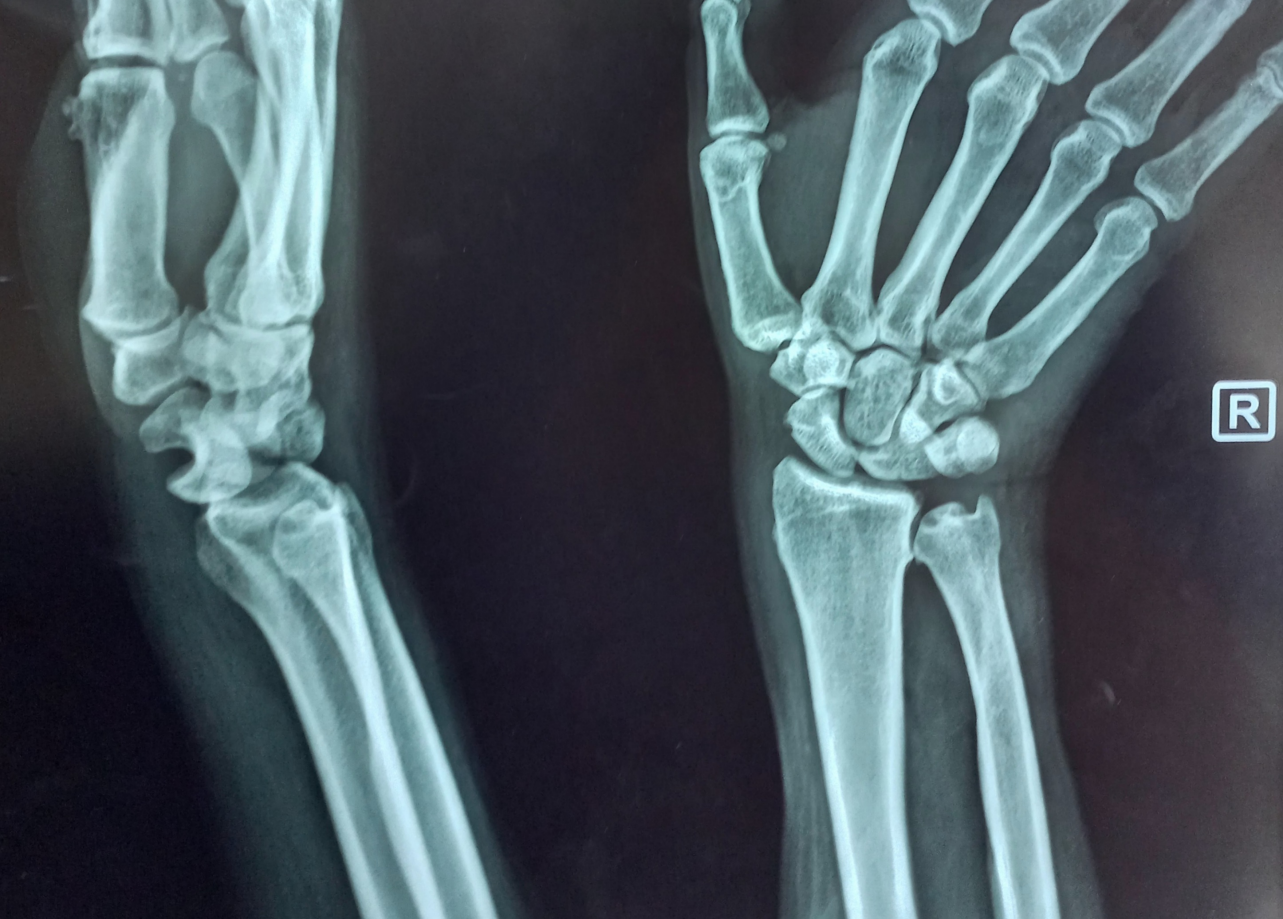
Preoperative posteroanterior radiograph showing disruption of Gilula’s arc, and lateral view demonstrating the “spilled teacup” sign.
